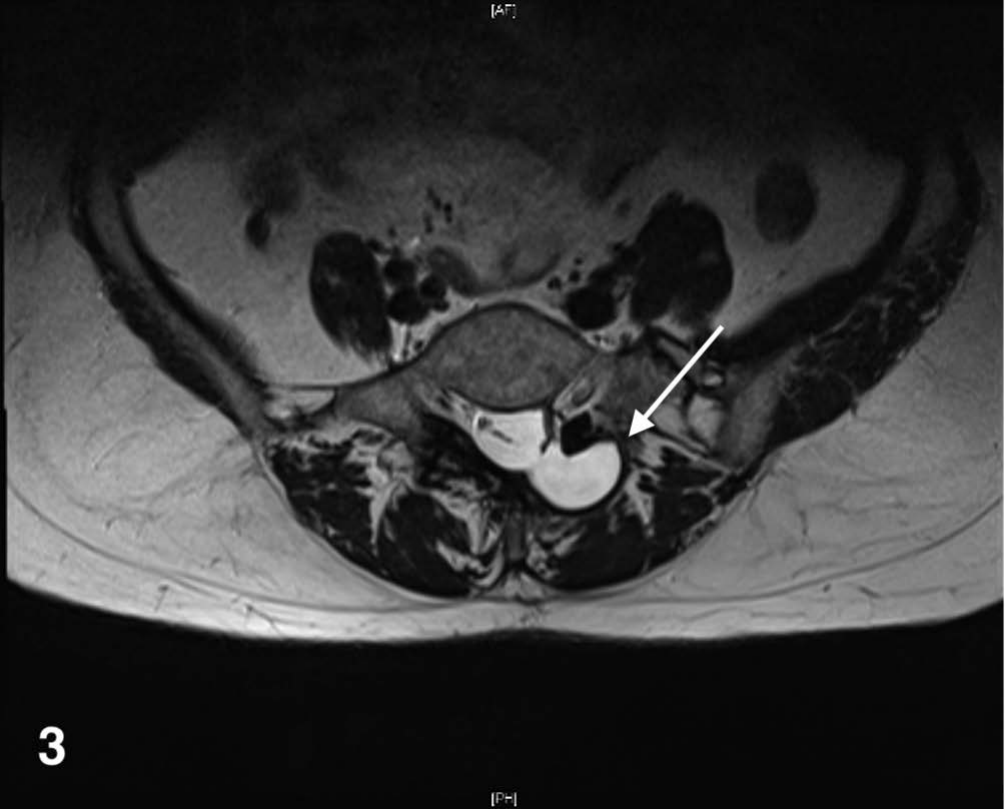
MRI horizontal plane of lumbar spine after UBE surgery. MRI = magnetic resonance imaging.
